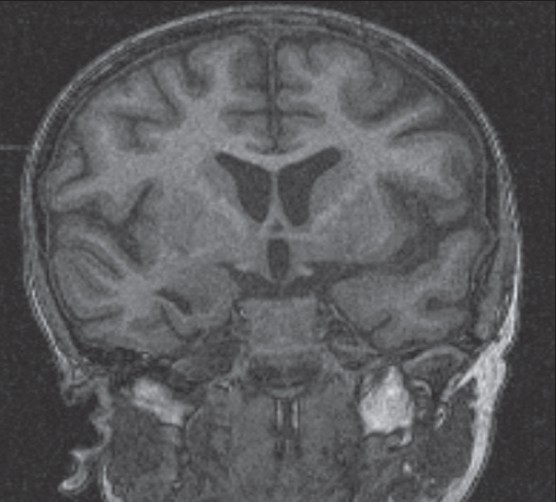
Thin section high resolution gradient echo MR image
